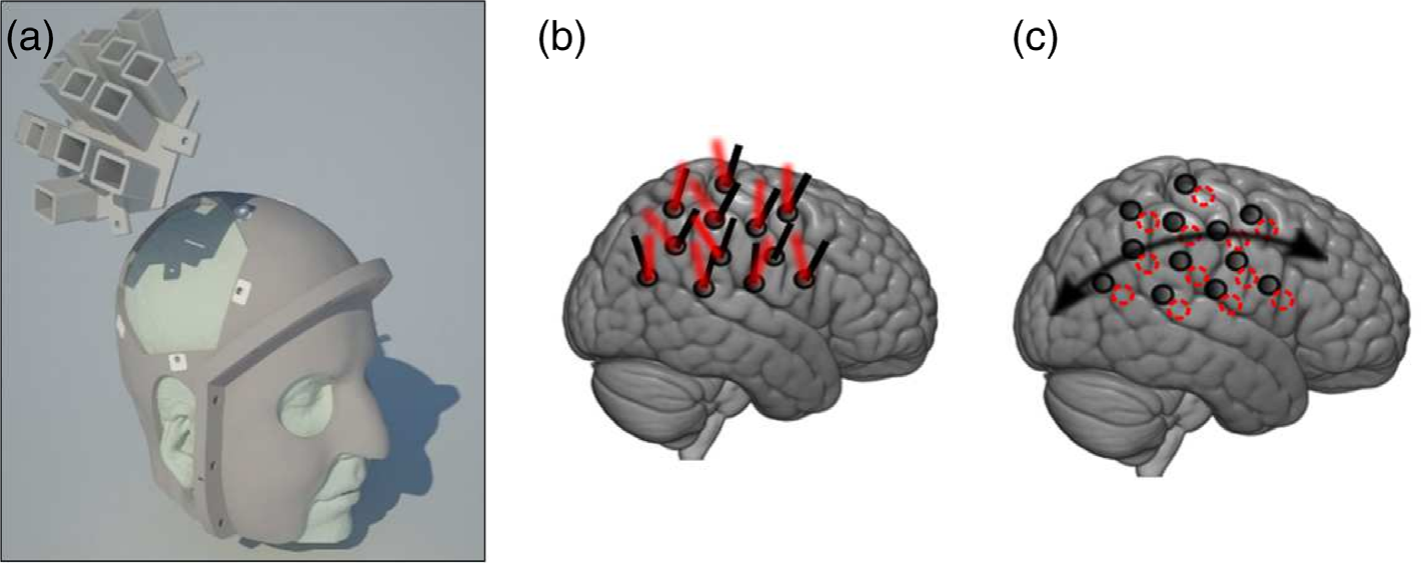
Head‐cast and array perturbations. (a) Digital model of the scanner‐cast. The cast is based on an individual MRI scan and designed to house the OPM sensors around the outer scalp surface. (b) Sensors were independently perturbed from their true orientation (black) by a fixed angle in random direction (red). (c) The rigid sensor array was displaced from its true position (black) to new positions (red) within an arc spanning −20 to 20 mm (and subsequently within a cube of 40 × 40 × 40 mm^3^) [Color figure can be viewed at http://wileyonlinelibrary.com]
